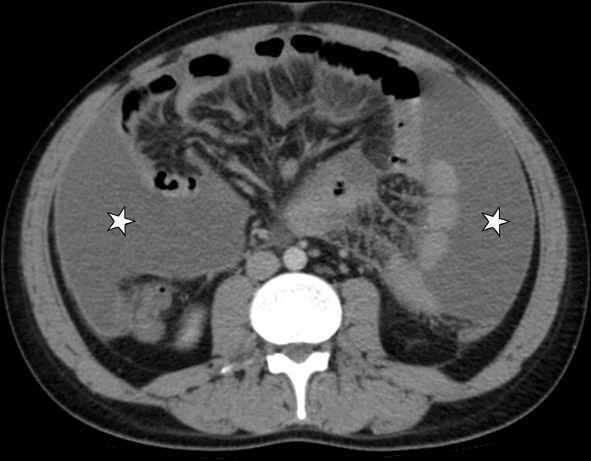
Transverse CT image with intravenous contrast of a 29-year-old male. Massive ascites is indicated in the abdomen cavity (stars). The peritoneum's thin linear contrast enhancement is also noted. Tuberculous peritonitis
